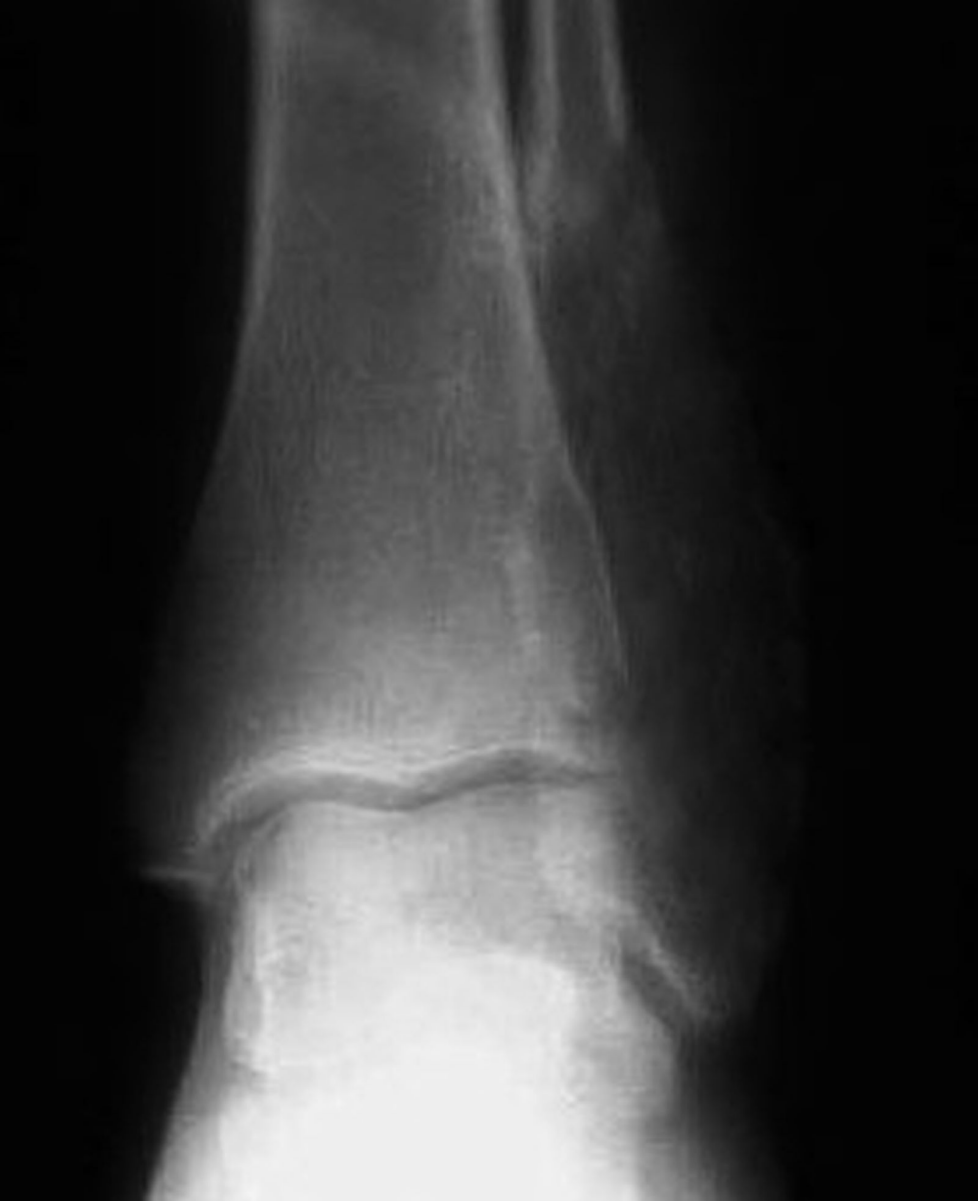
Plain radiograph anteroposterior view showing large expansile lytic lesion involving the distal end of fibula
